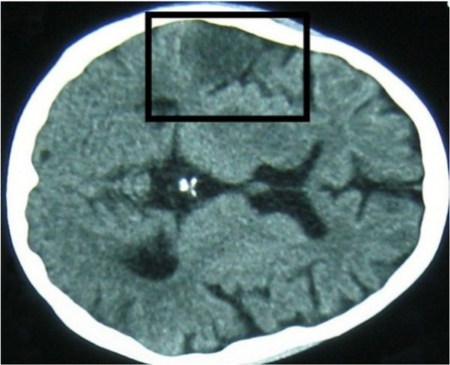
Computed tomography image: transverse section of the brain 1 month after the onset of illness. This section shows the infarctions of the right cerebral cortex
